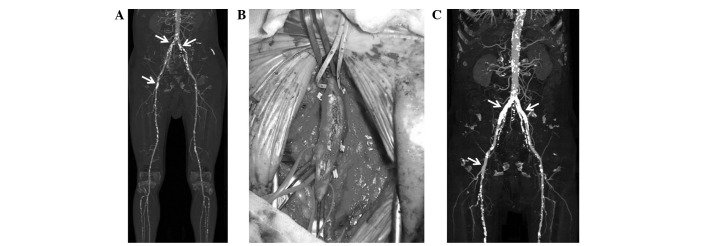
Combined femoral endarterectomy and bilateral iliac kissing stent. (A) Preoperative CTA showing a highly calcified lesion on the right CFA and bilateral common iliac arteries. (B) Image of the right CFA showing calcified plaques which were endarterectomized. (C) Postoperative CTA showing patent iliac stenting and a widely opened right common iliac artery. CTA, computed tomography-angiography; CFA, common femoral artery.
